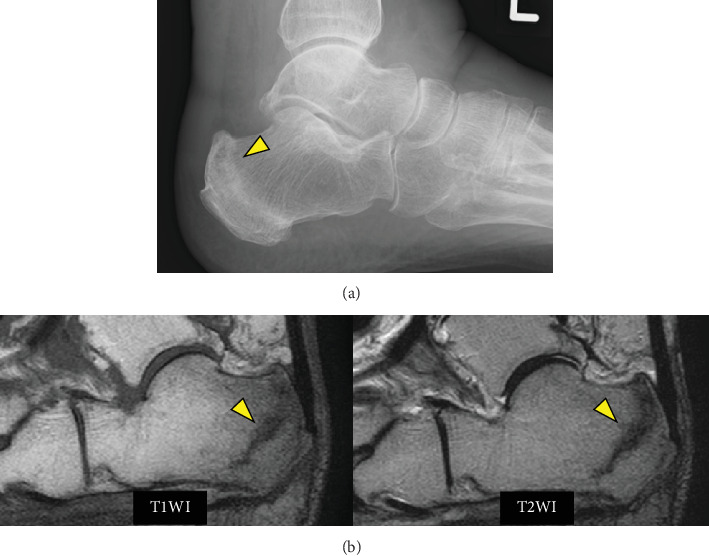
(a) Initial radiograph of the calcaneus taken 9 weeks following total knee arthroplasty, at symptom onset. The calcaneal posterior facet is displaced. (b) The sagittal view of the T1- and T2-weighted images shows an irregular fracture line in the calcaneus from the insertion of the plantar aponeurosis to the insertion of the Achilles tendon.
